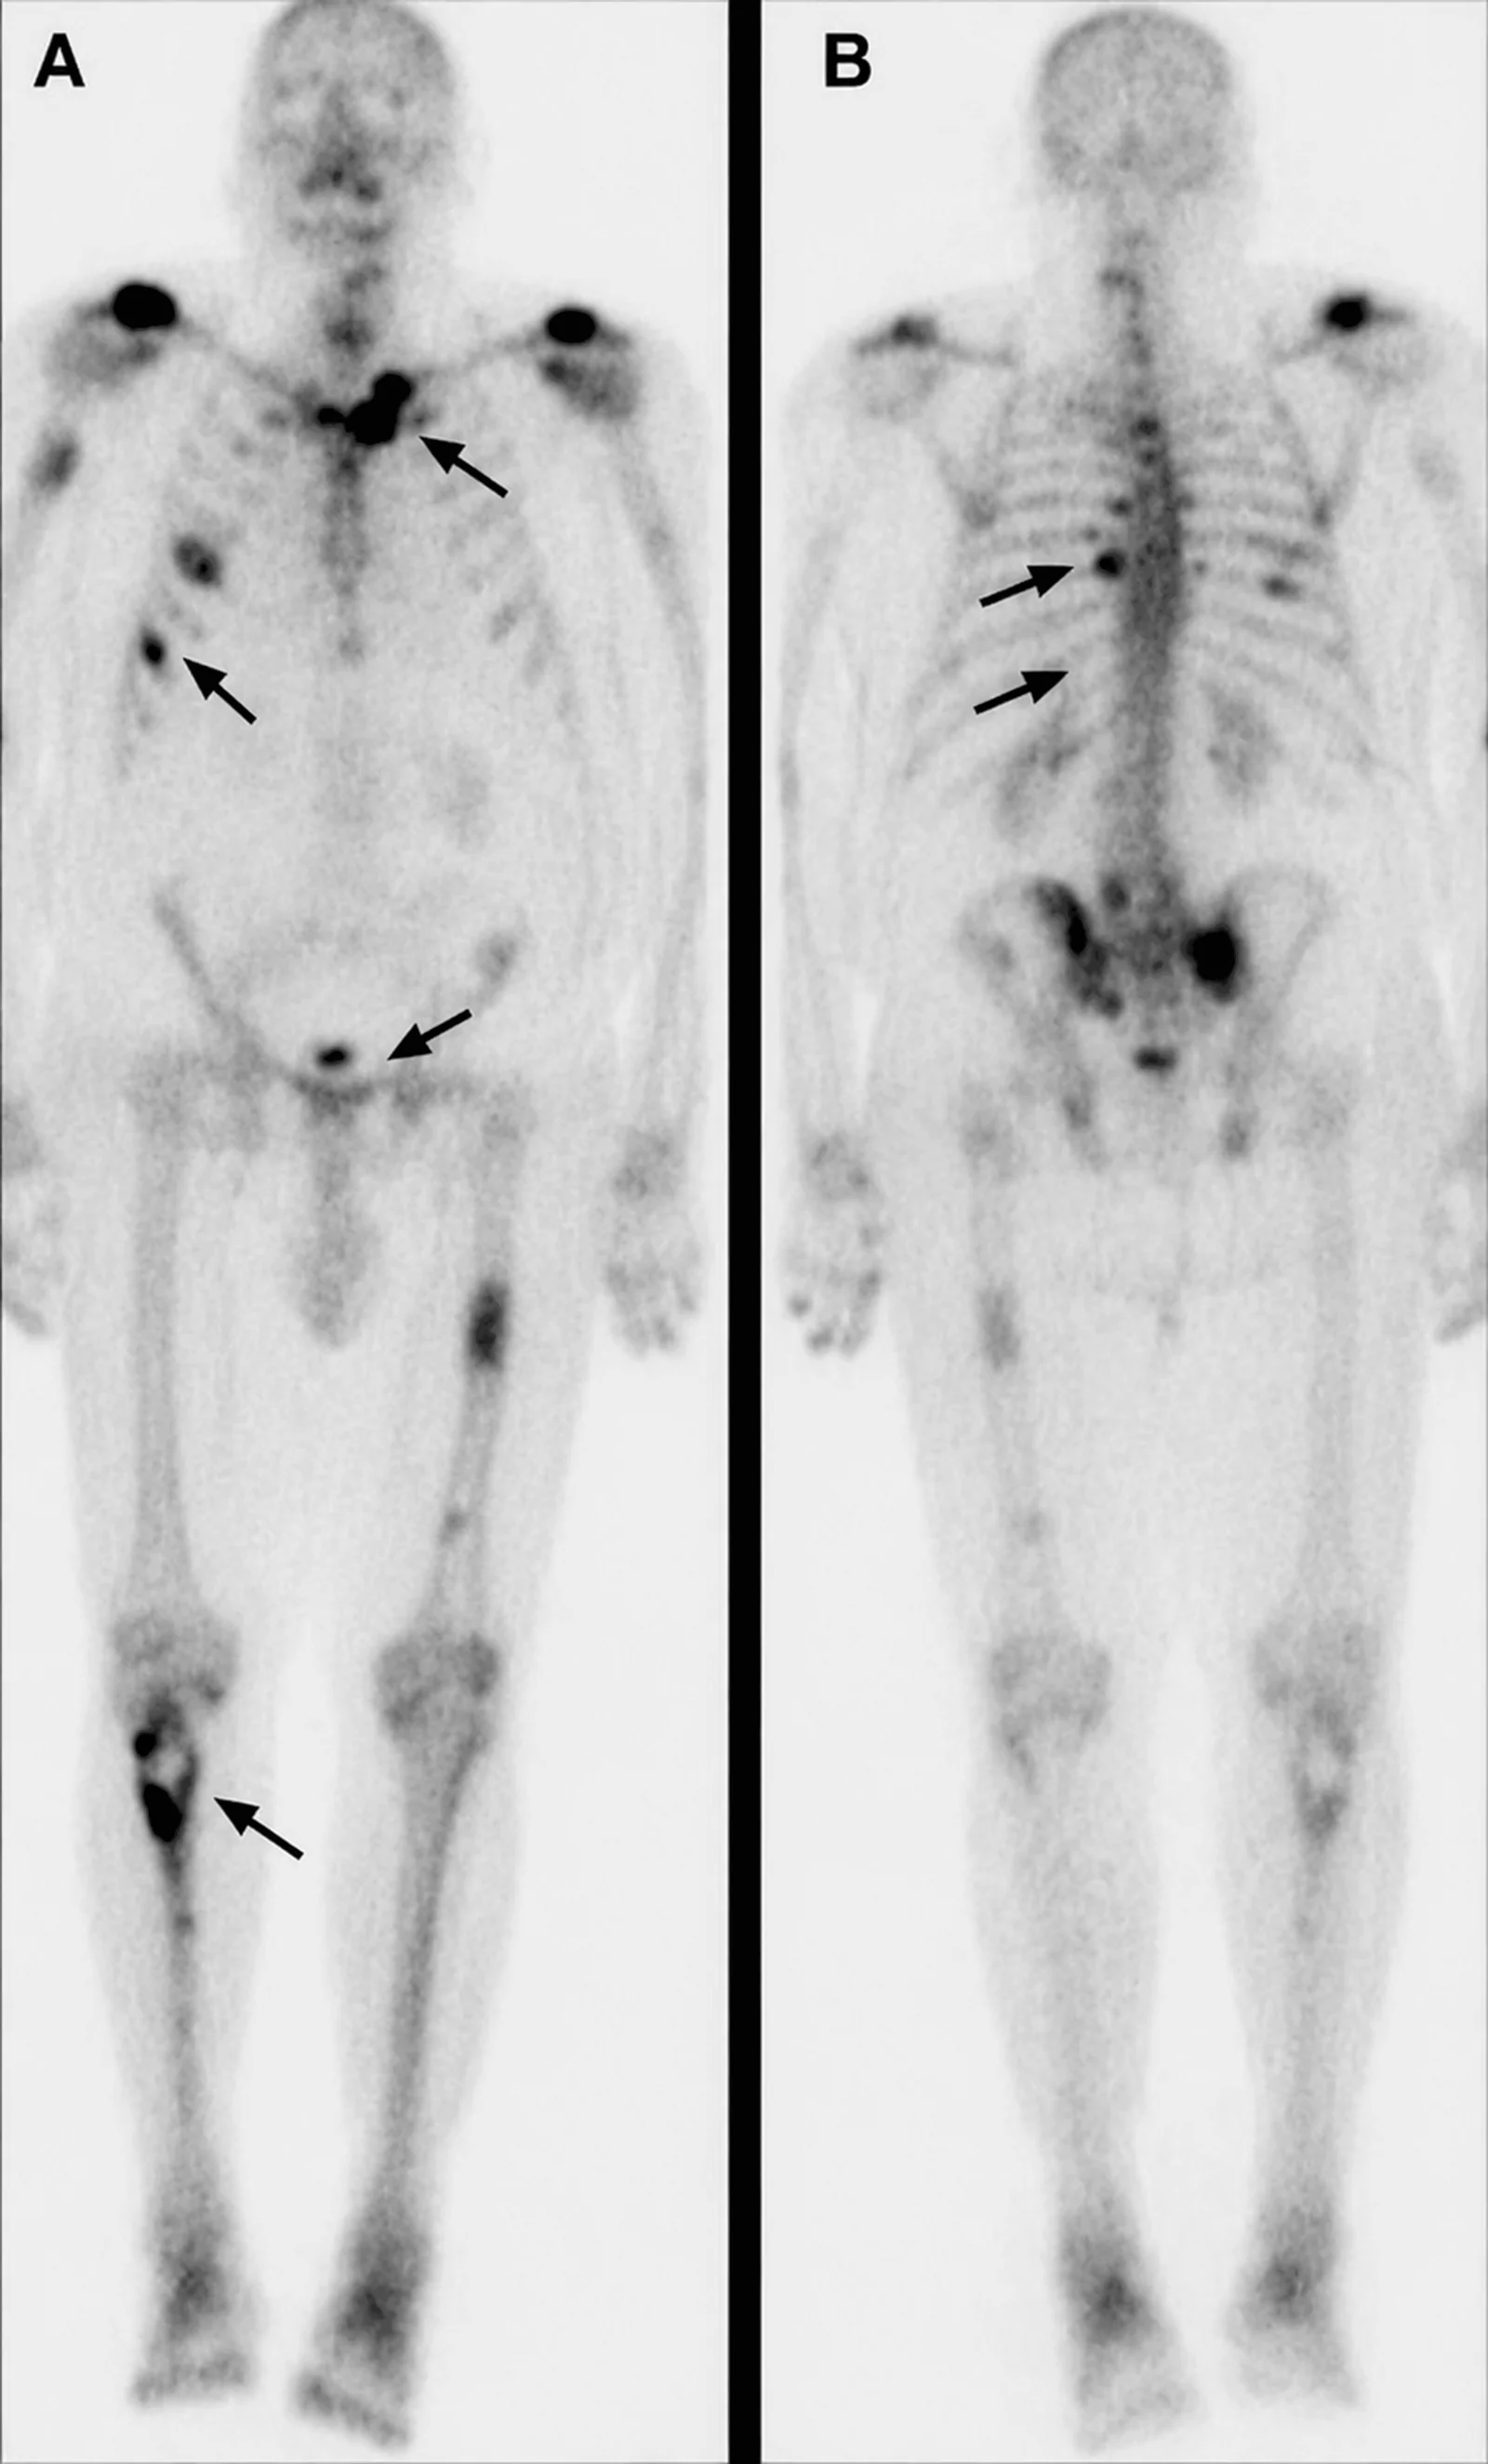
Whole-body bone scintigraphy in ferric carboxymaltose-induced hypophosphatemic osteomalacia. (A) Anterior view and (B) posterior view demonstrating multiple areas of increased tracer uptake involving the shoulders, clavicles, ribs, pelvis, and long bones, consistent with metabolic bone disease secondary to hypophosphatemia.
